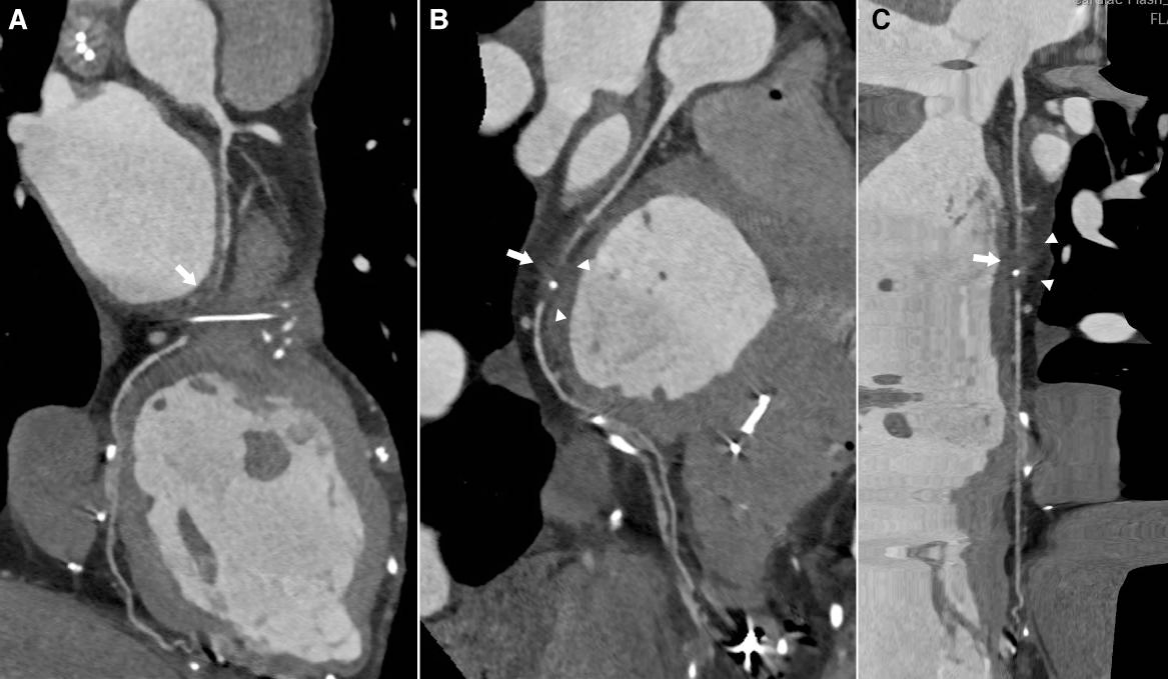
Curved (*A*, *B*) and stretched (*C*) multi-planar coronary computed tomography angiography reconstructions clearly show the segmental occlusion (arrows) of mid-left-circumflex artery due to extrinsic compression by a solid tissue (arrowheads in *B* and *C*) grown along the contiguous and abandoned epicardial lead.
